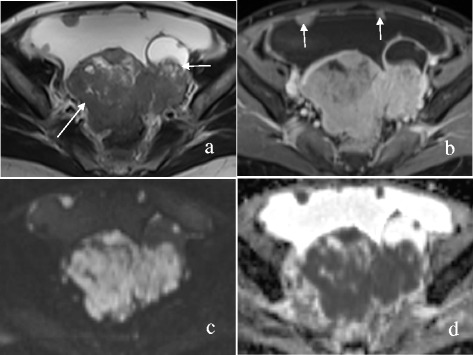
A 57-year-old woman with bilateral HGSC. Axial T2WI (a) demonstrates a solid mass (long arrow) in the right-sided ovary and a mixed cystic-solid mass (short arrow) in the left-sided ovary, which have an irregular shape. A large volume of ascites is observed. The solid components of the masses and peritoneal nodules (arrows) are enhanced markedly on contrast-enhanced T1WI with fat suppression (b), hyperintensity on DWI (c) and hypointensity on ADC map (d) with an ADC value of 0.759 × 10^−3^ mm^2^/s (left) and 0.782 × 10^−3^ mm^2^/s (right).
